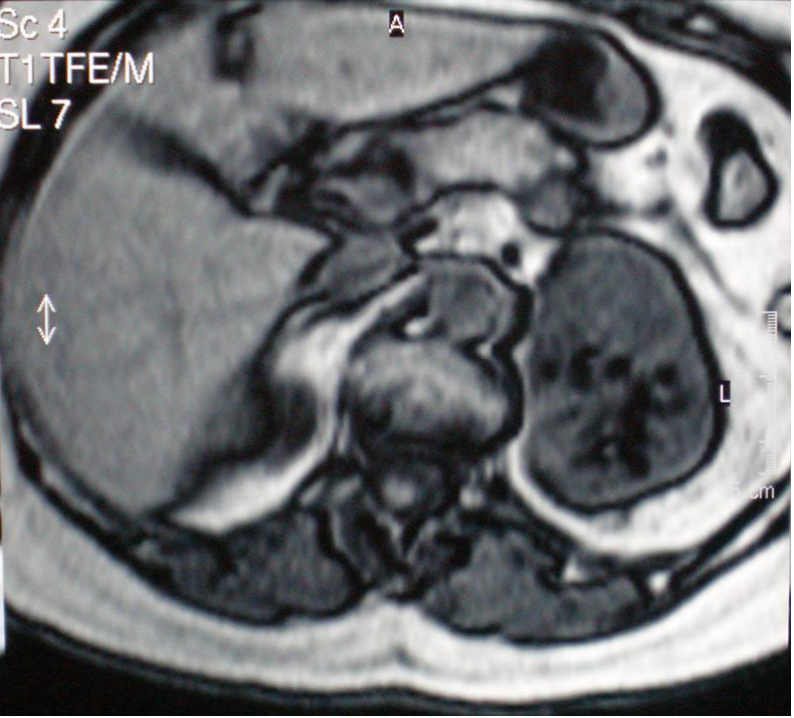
MRI scan of a left adrenal hemangioma demonstrating hyperintensity on T1-weighted image with a fat component.
